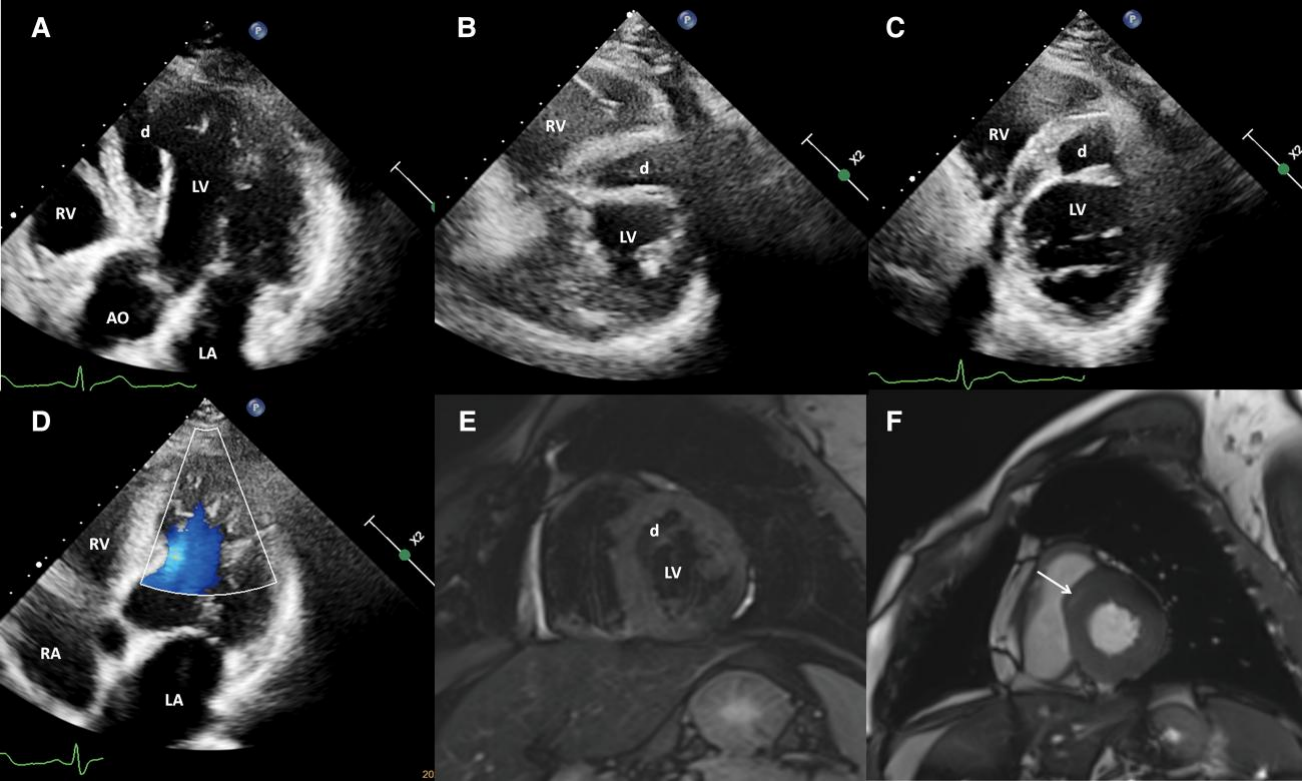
Echocardiography reveals a myocardial dissecting-like tear and rupture into the left ventricle in the interventricular septal and anterior left ventricular wall and apical regions (d) without haemodynamic obstruction between the left ventricular and the dissecting cavities (*A–C*) with mild reduced interventricular septal and apical contractility. There is a valve-like movement flap (*A*) between the left ventricle and the dissecting cavity without haemodynamic obstruction (*D*). Cardiac magnetic resonance reveals the myocardial dissection (d) (*E*) and the late gadolinium enhancement (white arrow) at the basal anteroseptal segment (*F*). AO, aorta; LA, left atrium; LV, left ventricle; RA, right atrium; RV, right ventricle.
